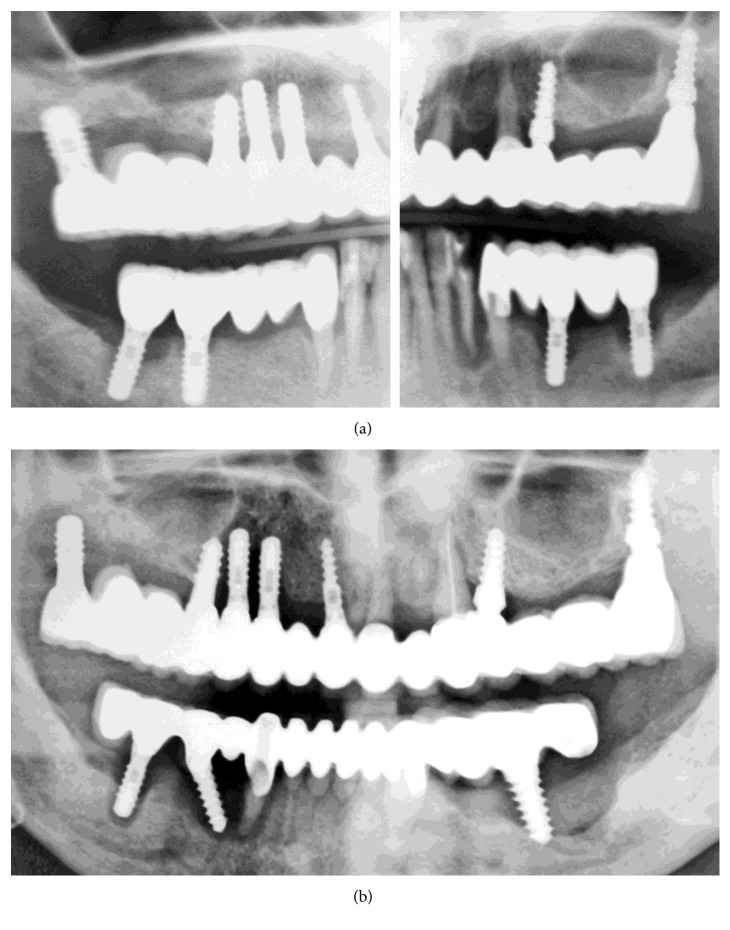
OPGs. (a) Further progression of bone loss on November 2009 (not modified radiograph taken during current treatment). (b) At initial examination in June 2015 (not modified radiograph taken during current treatment).
